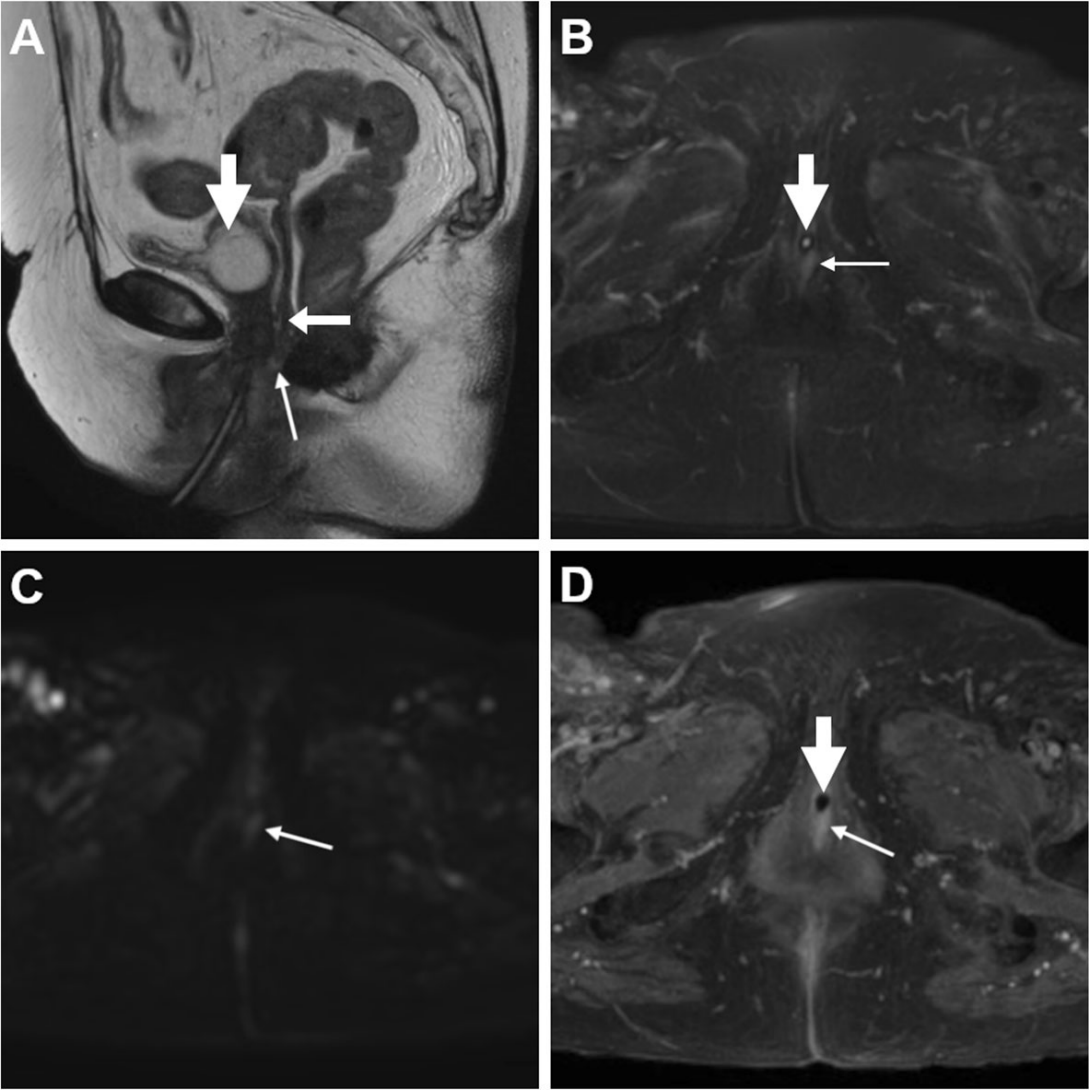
Urethro-VF in an elderly woman seen at MRI as subtle midline track (thin arrows) between the distal third of the vagina (arrow) and urethra containing Foley catheter (thick arrows), with high signal intensity on T2- (a), FS T2-weighted (b) and high *b* value DW (c) sequences, positive enhancement on post-gadolinium FS T1-weighted acquisition (d)
